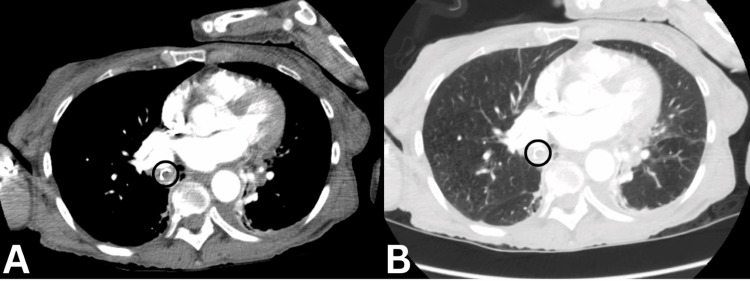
Computed tomography scan (axial view) showing 9-mm radiopaque body within the right lower lobe: (A) mediastinum window and (B) lung window
